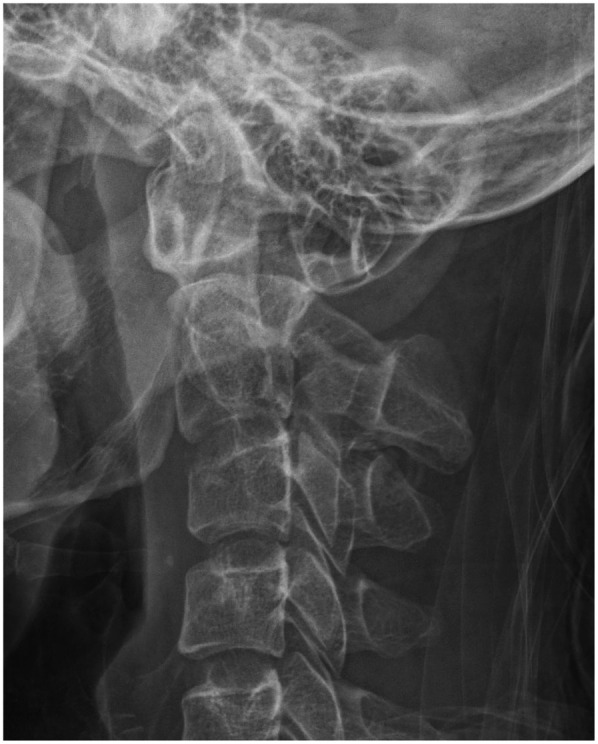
Preoperative lateral cervical radiographs. Preoperative cervical x-ray showed C2 Hangman fracture.
